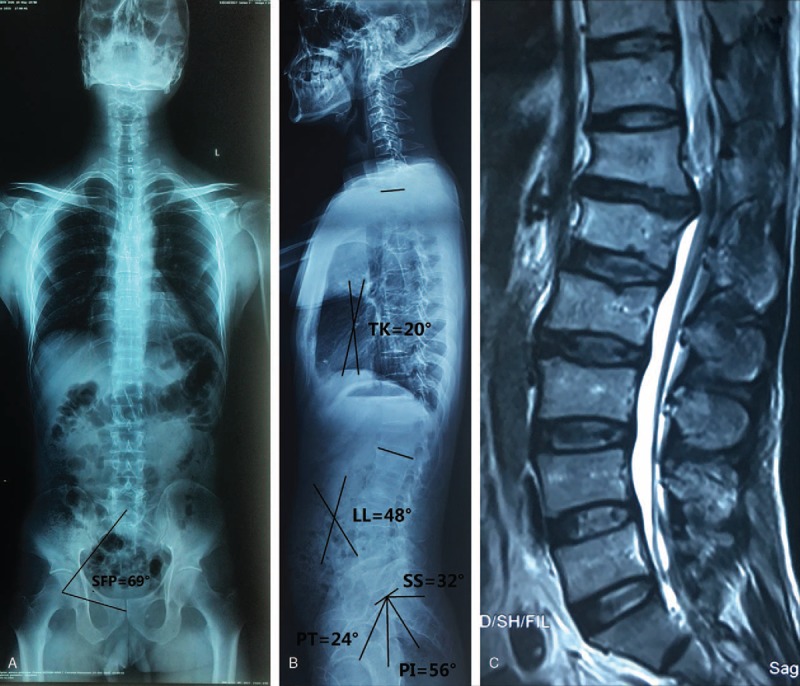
A male, 50 years old. SFP = 69°, PI = 56°, PT = 24°, SS = 32°, LL = 48°, TK = 320°. (A) A positive full spine X-ray; (B) lateral full spine X-ray; (C) lateral lumbar MRI showed disc herniation at T12/L1 level. LL = lumbar lordosis, PI = pelvic incidence, PT = pelvic tilt, SFP = sacrum-femoral-pubic symphysis, SS = sacral slope, TK = thoracic kyphosis.
